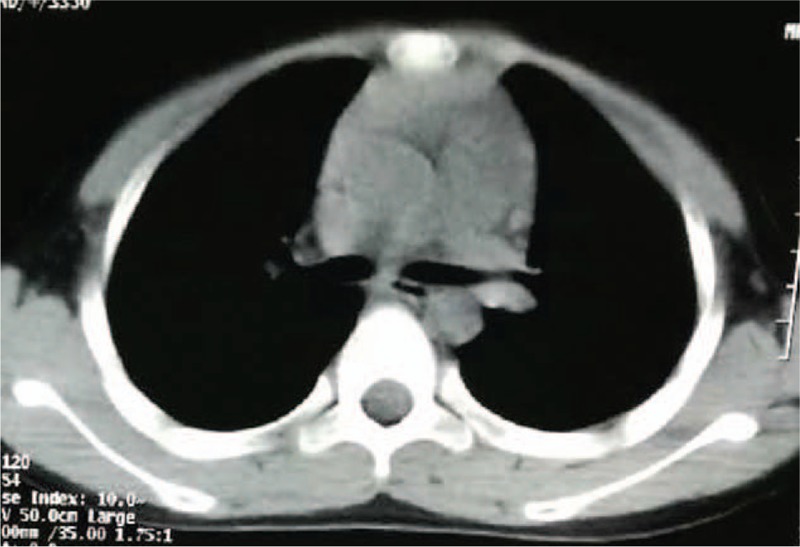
Postoperative CT scan of the chest showed negative for tumor recurrence.
